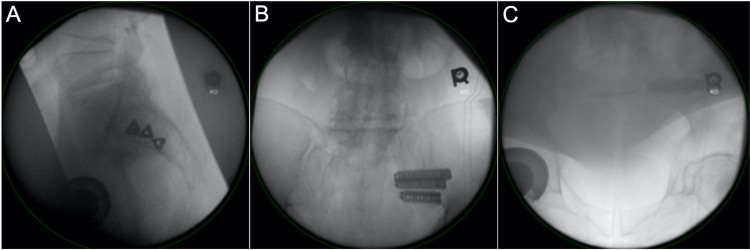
X-ray fluoroscopy of the pelvis showing final implant placement: (2A) lateral view, (2B) an outlet view, and (2C) an inlet view.
